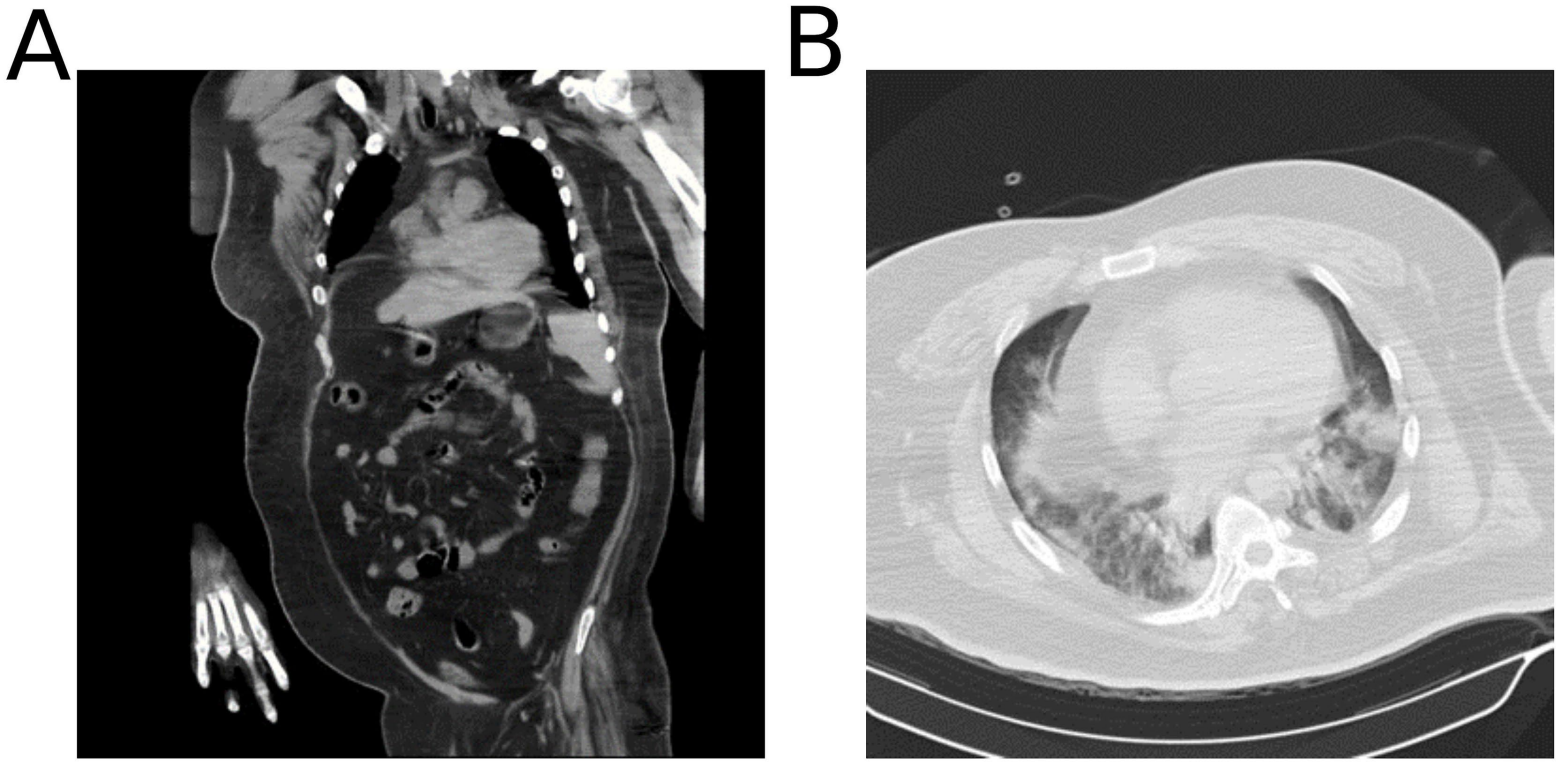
Chest radiography revealed pneumonia. (A) Chest radiograph and (B) computed tomography.
